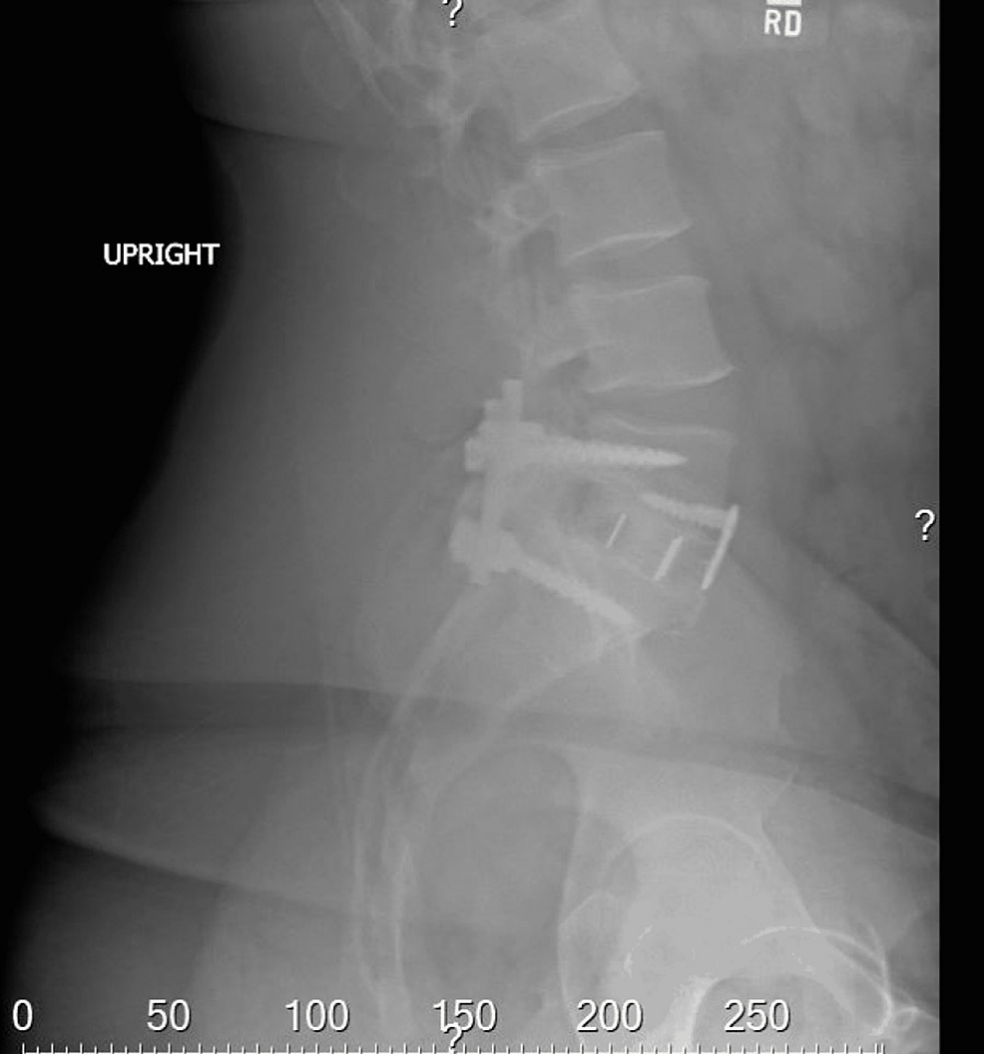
Lateral x-ray of the lumbar spine.
